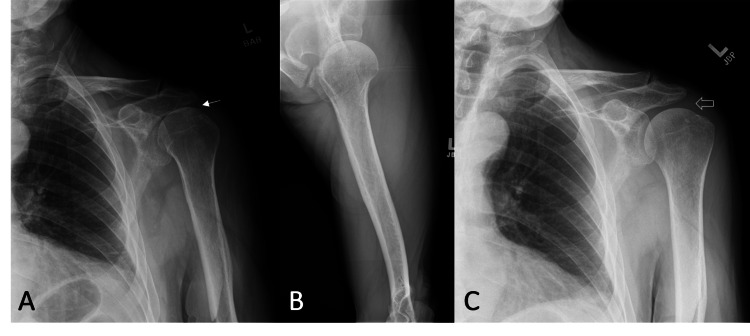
Left anteroposterior (A) and axillary (B) views revealed mild proximal humeral head migration (arrow) with mild glenohumeral and moderate acromioclavicular joints degenerative joint disease. Proximal humeral head migration (open arrow), a radiographic sign of rotator cuff arthropathy, was not present a year earlier (C).
